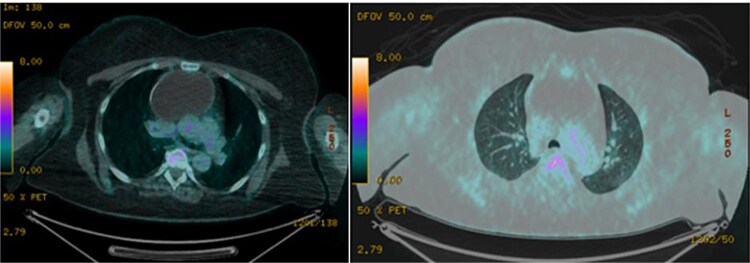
Axial images of PET/CT demonstrating a photopenic mass in the anterior mediastinum measuring 7.0 × 9.4 cm.
